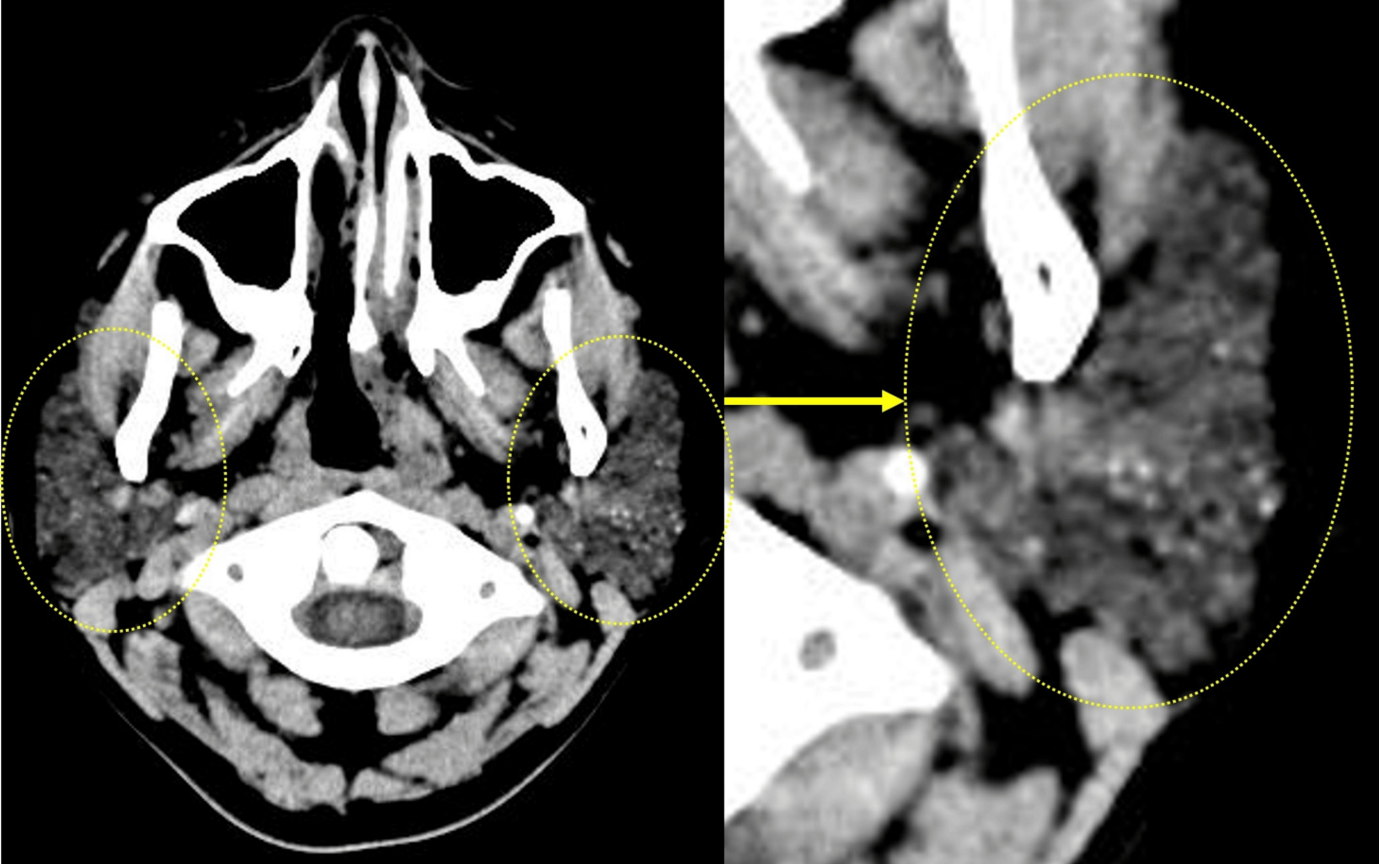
Computed tomography (CT) scan of the bilateral parotid glands A plain head CT scan shows multiple microcalcifications within the bilateral parotid glands, predominantly on the left side.
